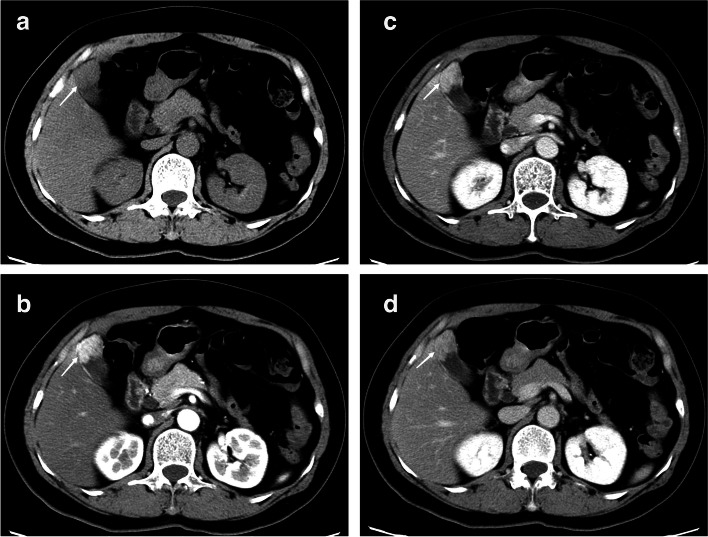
A 62-year-old women patient with gallbladder polypoid lesions (gallbladder carcinoma), which was wild base. a Plain phase CT values was 52 HU, b artery phase CT values was 102 HU, c portal venous phase CT value was 95 HU, and d delayed phase CT value was 87 HU
